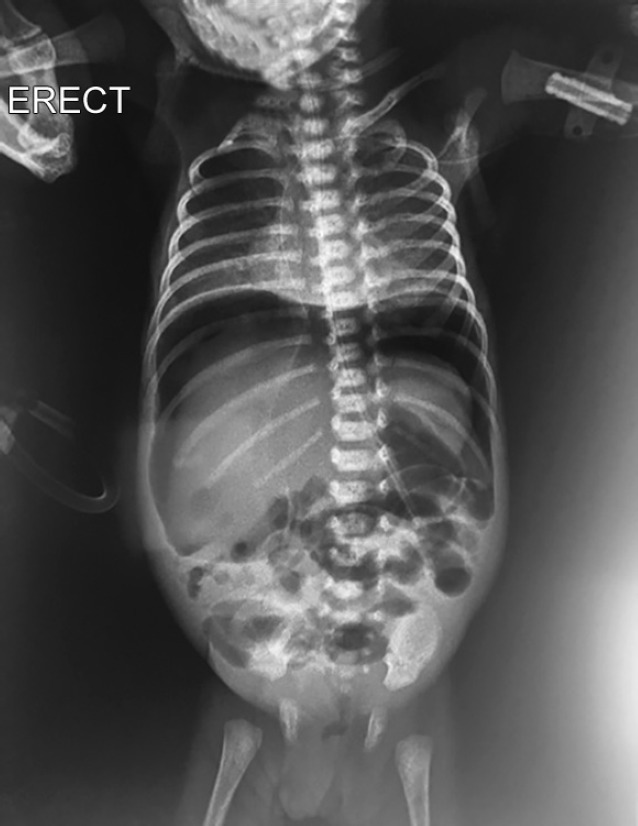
X-ray picture of pneumoperitoneum after which ileostomy was done
